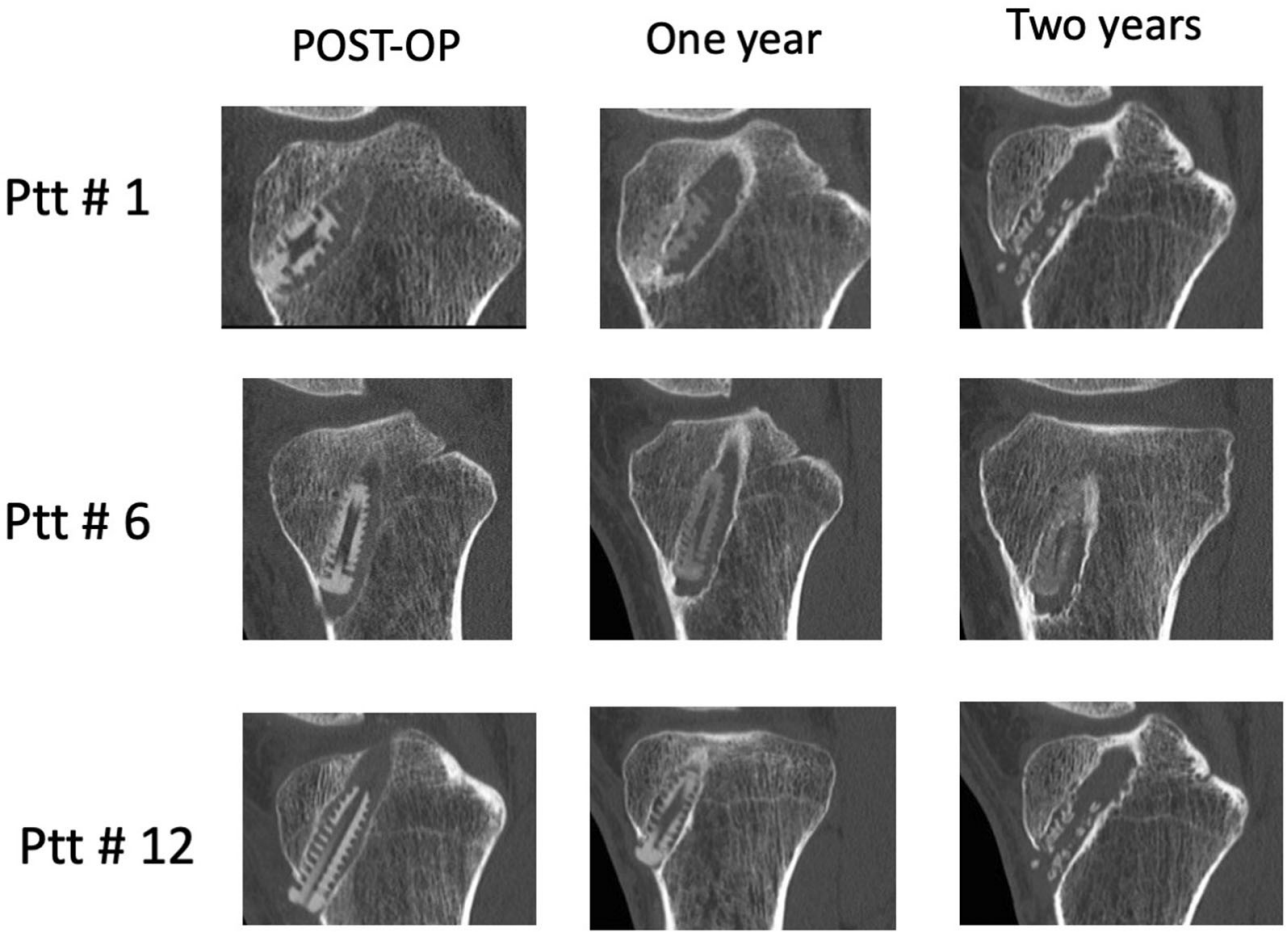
Three examples of the screw resorption process with representative computed tomography scan images in the immediate postoperative period and at the one‐ and two‐year follow‐ups.
